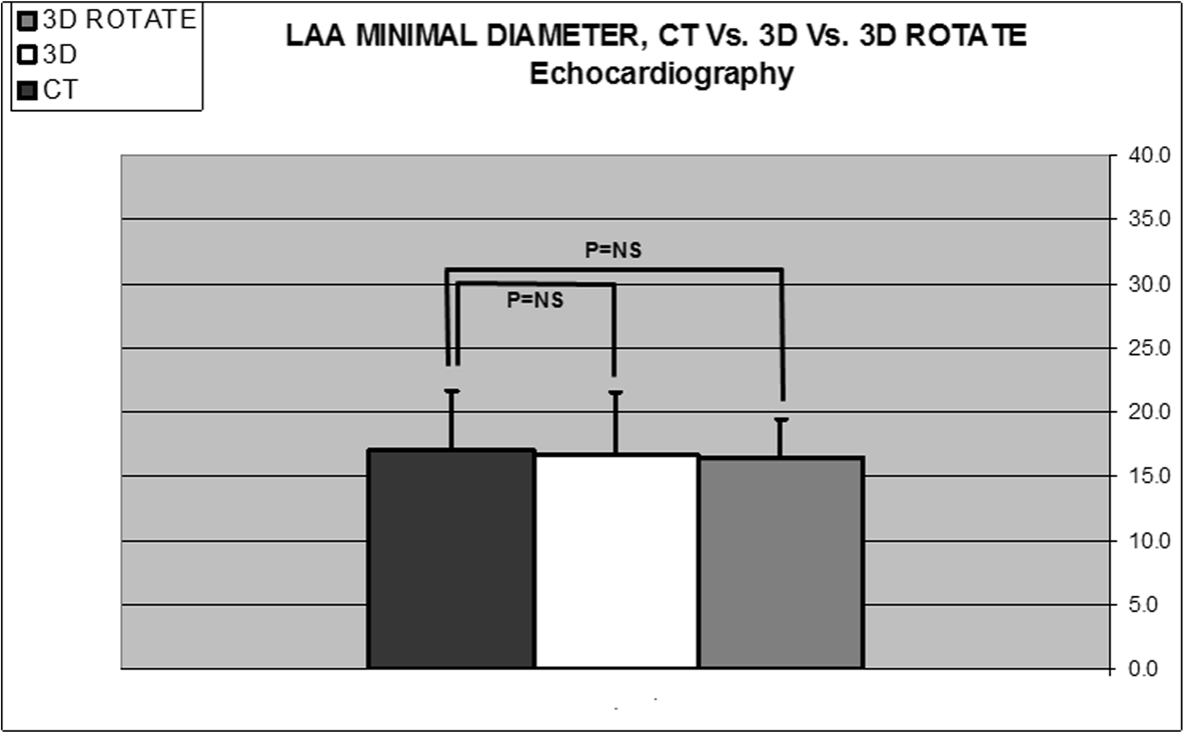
Histogram comparing LAA minimal diameter using different imaging methods (*n* = 41). Comparison between computed tomography (CT) (17.0 ± 4.4 mm), direct Real-Time 3-Dimensional Transesophageal Echocardiography (3D) (16.7 ± 3.3 mm), and Rotational 3DTEE (3D Rotate) (16.4 ± 3.4 mm), (*p* = NS for all)
